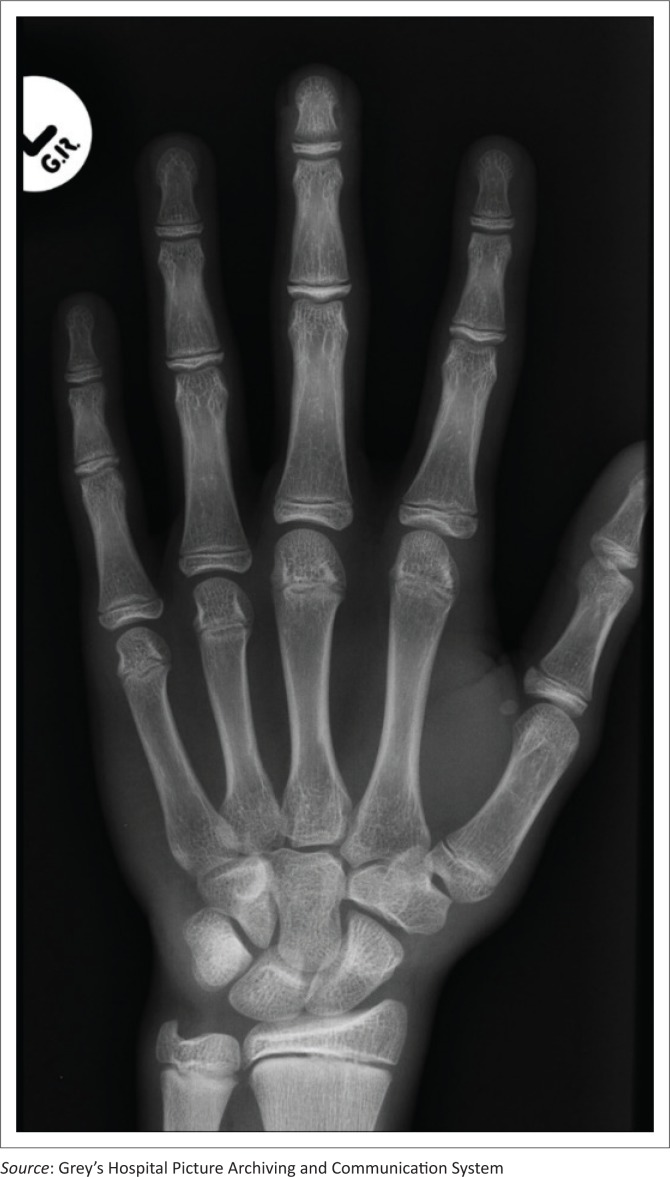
Digital hand-wrist radiograph (left) of a male subject with a chronological age of 15 years 10 months was matched to the GP standard image of a 14-year-old subject by both readers.
